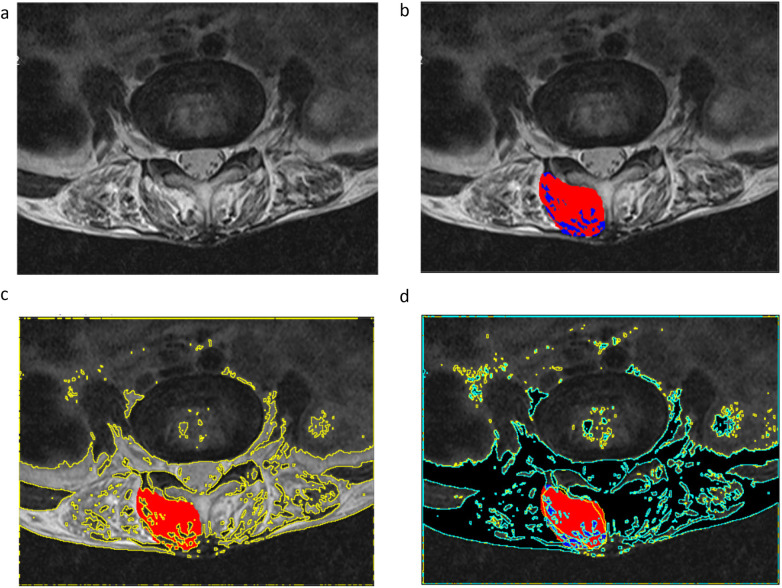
All images (including Figure 1a) are of the lumbar MRI scans of a patient at our hospital prior to treatment. ImageJ software was used to measure the lumbar multifidus muscle (LMM) in the corresponding segment. The blue and red areas marked in Figure 1b represent the LMM, while the white and dark blue areas in Figures 1c and 1d represent fat.
